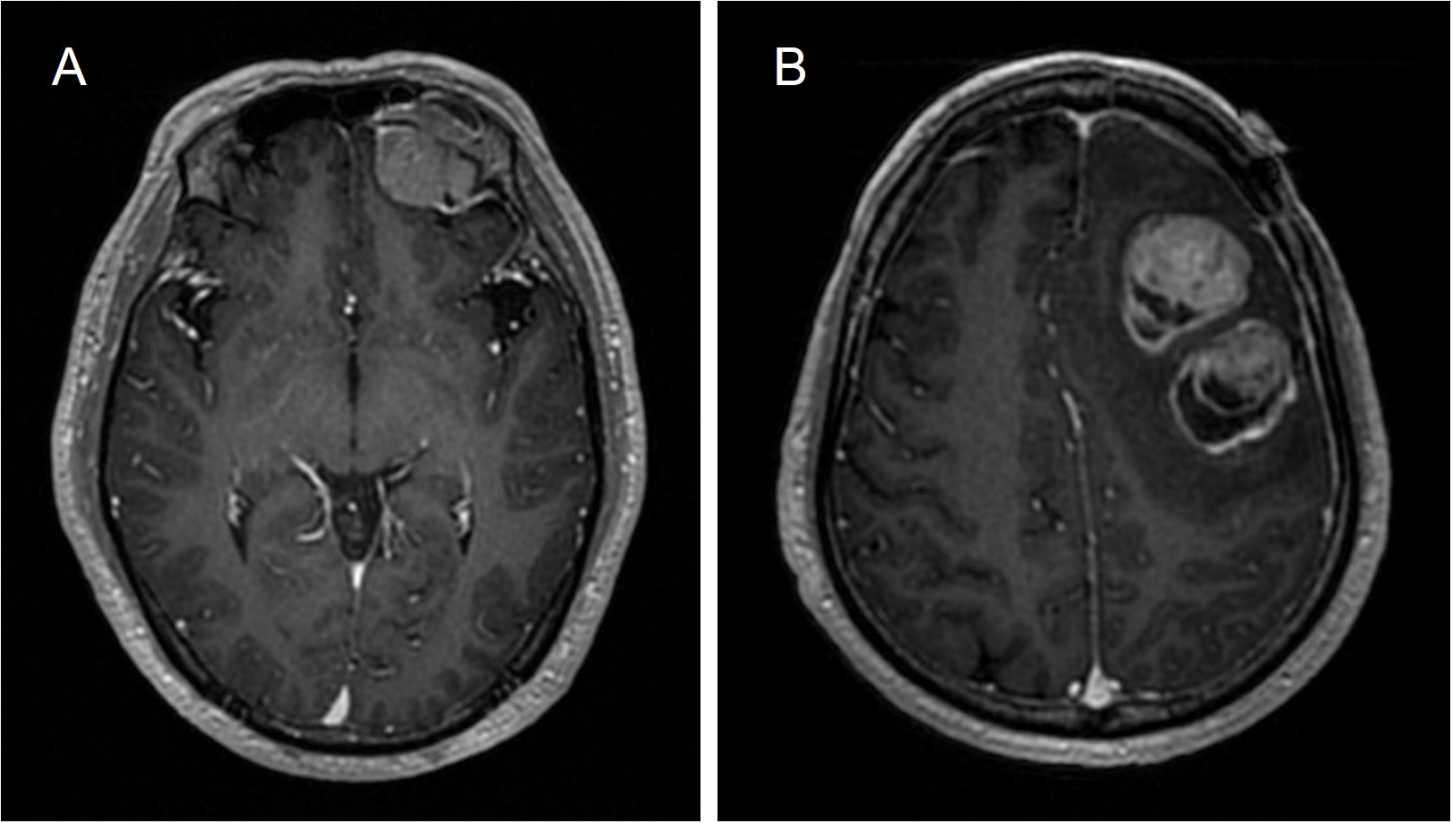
Brain MRI. (A) Initial primary tumor. A heterogeneously enhancing tumor on the left frontal lobe (32 × 29 × 31 mm^3^) with an associated mass effect. (B) Metastasis. Three well-defined, heterogeneously enhanced tumors in the left upper frontal lobe with found (39 ×31 ×42 mm^3^, 34 ×34 × 31 mm^3^, 13 ×8 × 14 mm^3^) with associated mass effect, with surrounding edema.
